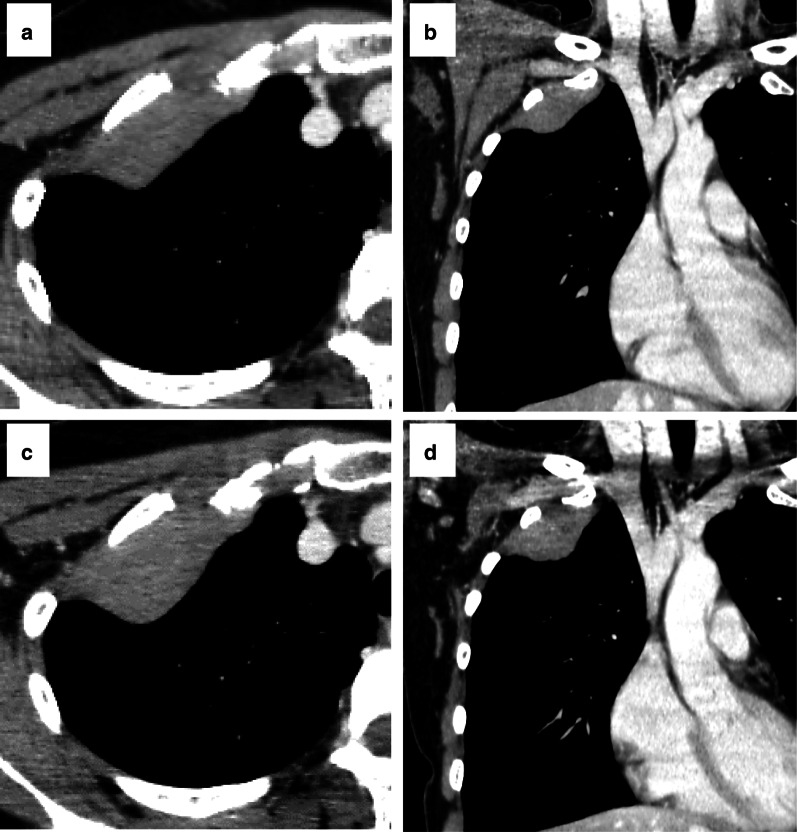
CT at the first visit. a Axial view. b Coronal view. There was a limbic smooth mass in extensive contact with the right chest wall. It had extended to the first and second intercostal area. No invasion to the subclavian vessels or brachial plexus was observed. CT two month after the first visit. c Axial view. d Coronal view. The tumor showed growth without invasion to surrounding tissues
